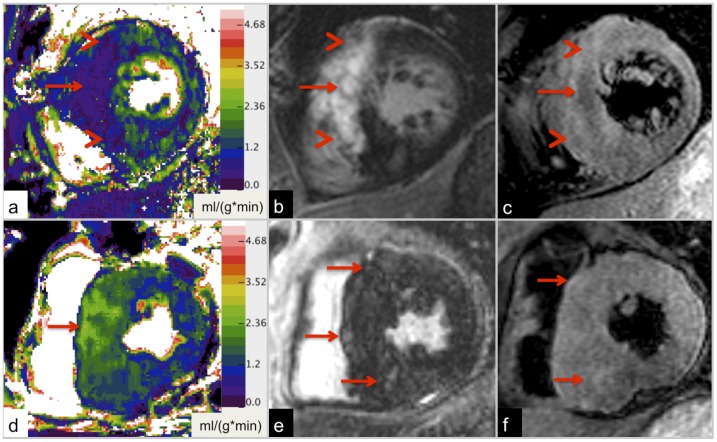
Examples of different perfusion patterns in HCM. 21-year-old patient with non-obstructive HCM (LV outflow gradient 7 mmHg). Large area of intense, well-defined LGE in the anterior septum and the anterior wall (b) with corresponding hypo-perfusion (relative MBF  = 33.5%; a). T2-weighted imaging depicts low signal in the central area of the lesion, suggesting macroscopic, chronic fibrosis/ scar tissue (red arrow), and adjacent high signal, indicating edema (arrow head, c). Maximum LV wall thickness is 31 mm. Findings of a 46-year-old HCM patient with an LV outflow gradient of 11 mmHg are given in d-f. Resting MBF of the interventricular septum is focally increased to 145% (d). In this area patchy and diffuse LGE with relatively low signal intensity (e) and patchy edema on T2-weighted images (f) are visible (red arrows). Maximum LV wall thickness is 33 mm.
